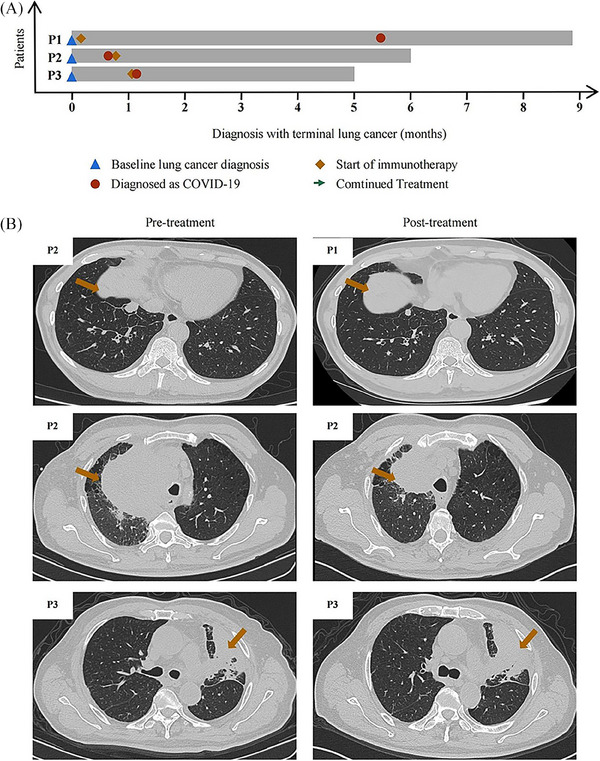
(A) Swimmer's plot of disease course and immunotherapy after COVID‐19 infection in the three patients (P1–P3). (B) Computed tomography of the chest of P1–P3 before and after immunotherapy. Brown arrows indicate pulmonary tumors. P1, patient 1; P2, patient 2; P3, patient 3.
